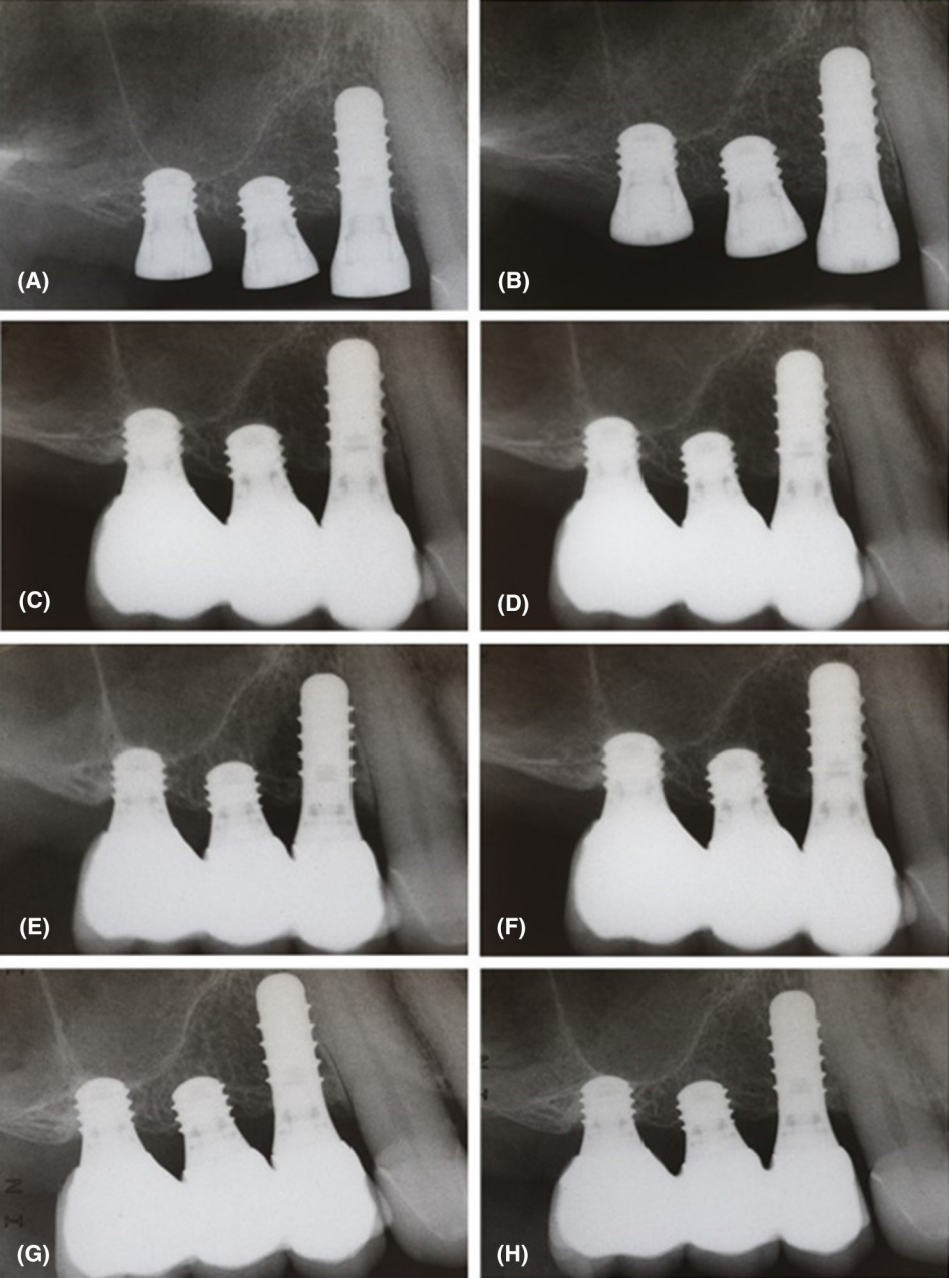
Radiographic examination of implants: (A) immediately after insertion, (B) 6 months after insertion, (C) immediately after crown cementation, (D) at the 1‐year follow‐up visit, (E) at the 2‐year follow‐up visit, (F) at the 3‐year follow‐up visit, (G) at the 4‐year follow‐up visit, (H) at the 5‐year follow‐up.
